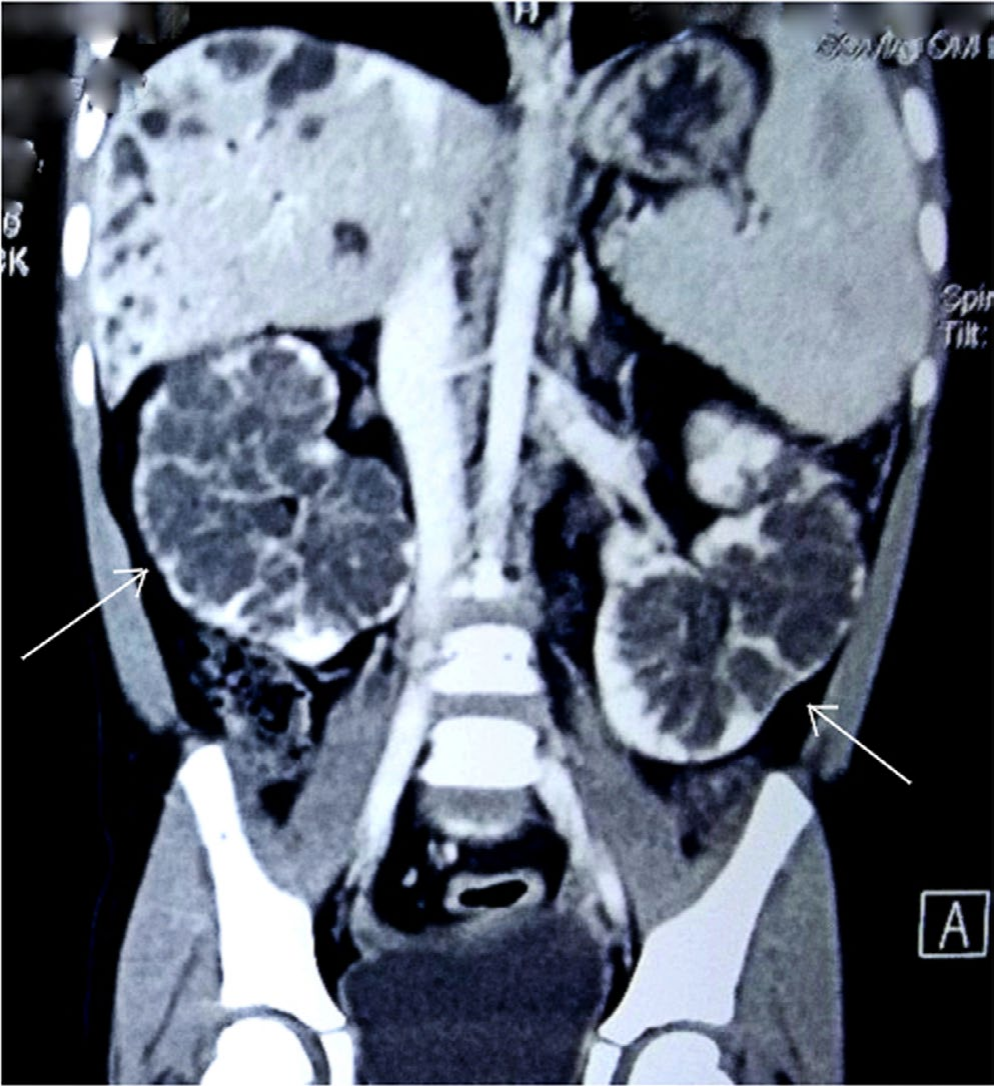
CT scan (coronal view) Multiple variable‐sized cystic lesions are seen (arrows) involving all poles of both kidneys. No calcification, septation, or enhancing solid components seen within the cyst
